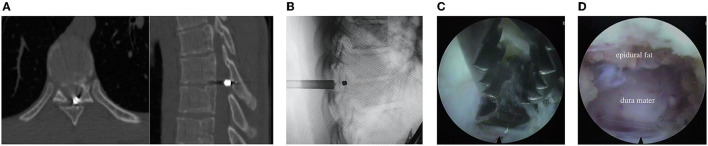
(A) CT scans of the thoracic spine (explained in the text); (B) Radiography of the working port and bullet; (C,D) Endoscopic stage of the surgery (described in the text).
